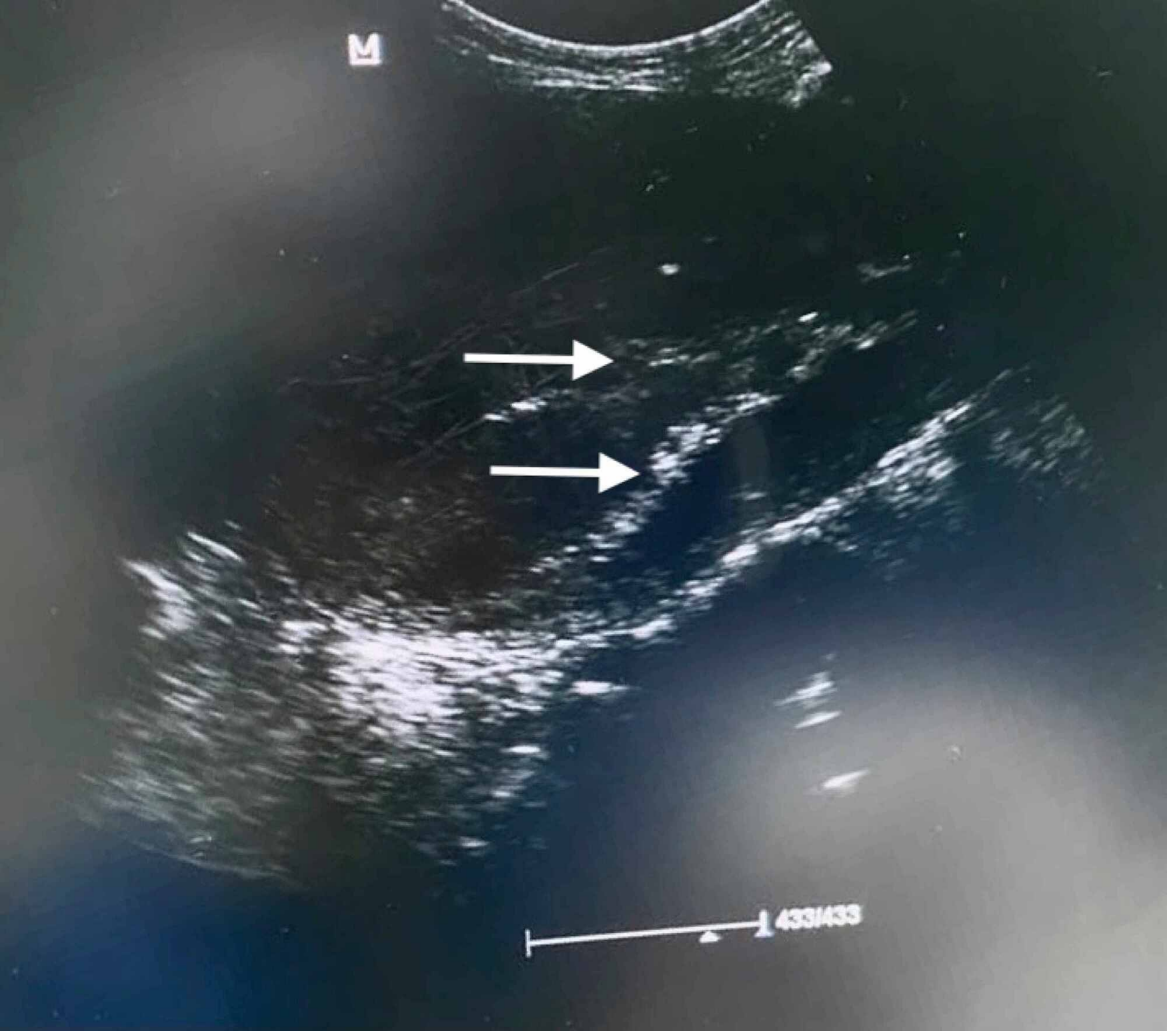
Ultrasound showing two endometrial linings.
